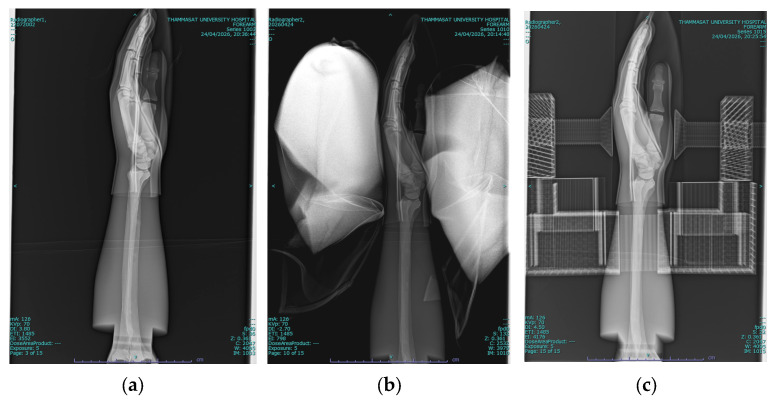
Representative radiographic images from three different positioning techniques. (a) Adhesive tape fixation technique. (b) Sandbag support technique. (c) 3D-printed wrist immobilization device.
